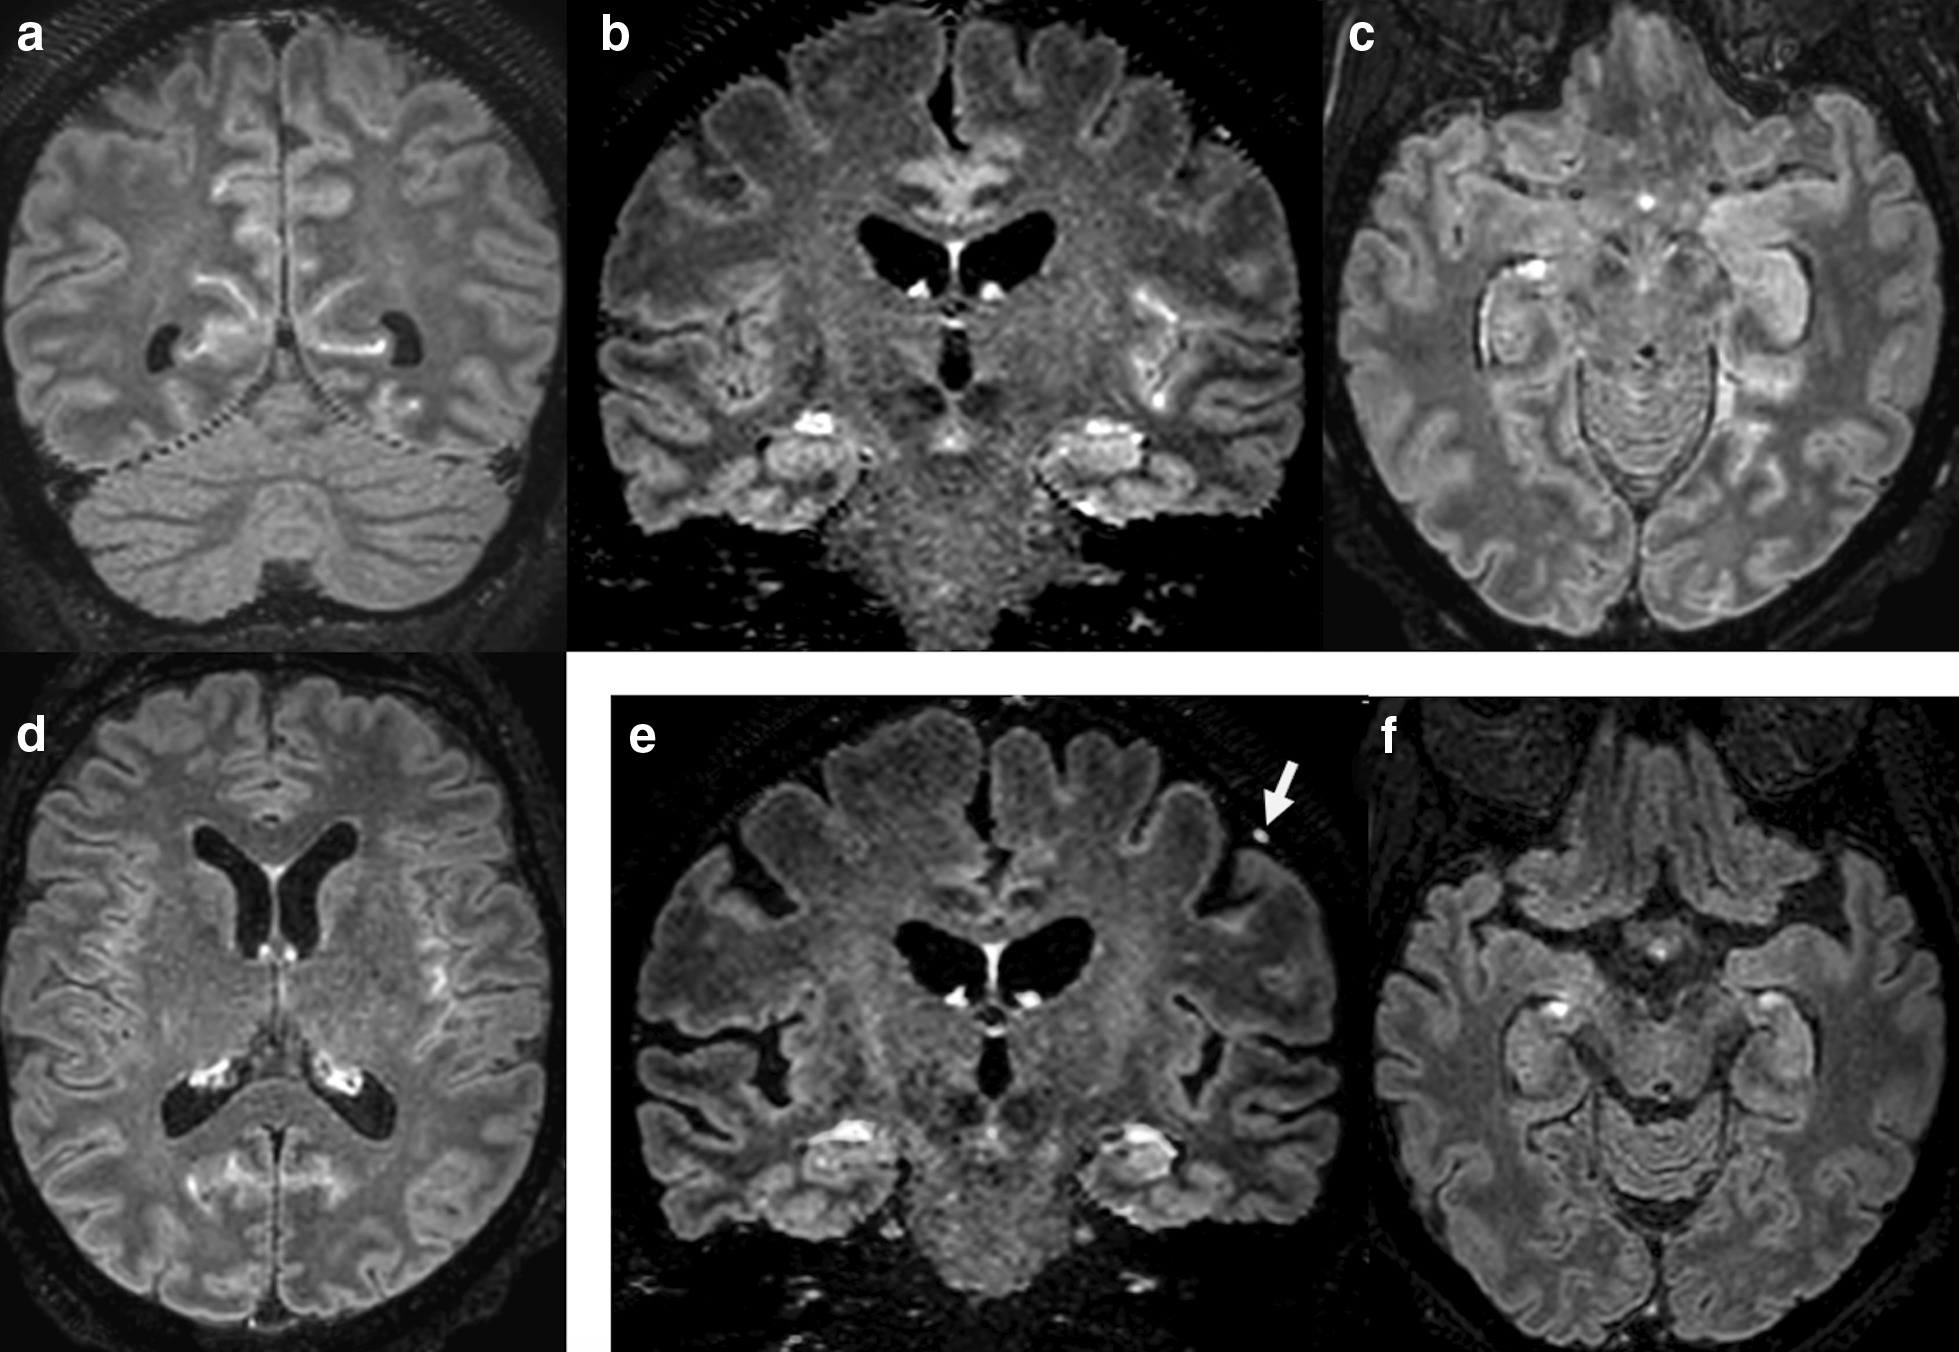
Brain MRI. Initial coronal (a, b) and axial (c, d) fluid-attenuated inversion recovery postcontrast MRI displaying hypersignal or enhancement of meninges, cortical and subcortical regions spread over the insula, the cingula, the medial part of the occipital areas and the internal part of the left temporal lobe. 10-week follow-up MRI (e, f) demonstrates complete disappearance of leptomeningeal and cortical FLAIR hyperintensities and enhancements, except for a subtle left-sided residual nodular leptomeningeal enhancement (arrow) adjacent to a cortical vein
